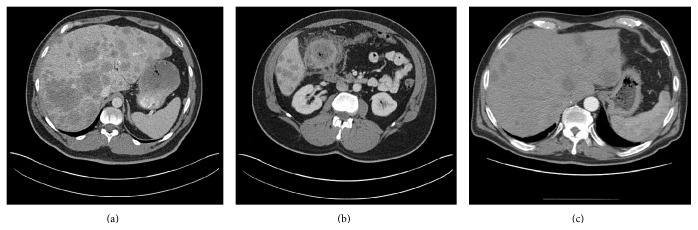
(a) CT scan of the abdomen showing innumerable liver metastases in a patient (Case 1) with colorectal cancer (a) and the primary mass in the ascending colon (b). Image (c) shows a similar scan with multiple liver metastases in another patient (Case 2) with mCRC.
